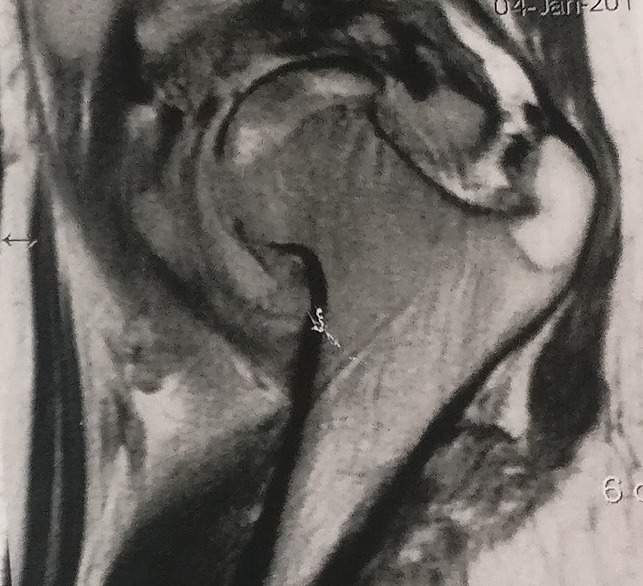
Radiograph showing the PDW T1 Sagittal corroborating the findings in other sections.
